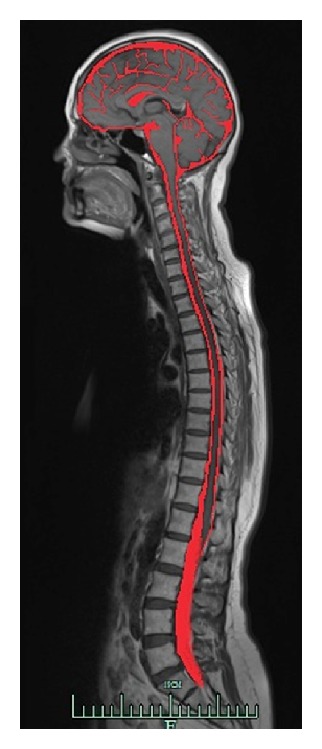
The MRI sagittal neural tube section. The cerebrospinal fluid surrounding the brain and the spine within subarachnoid space is red-colored on this image.
